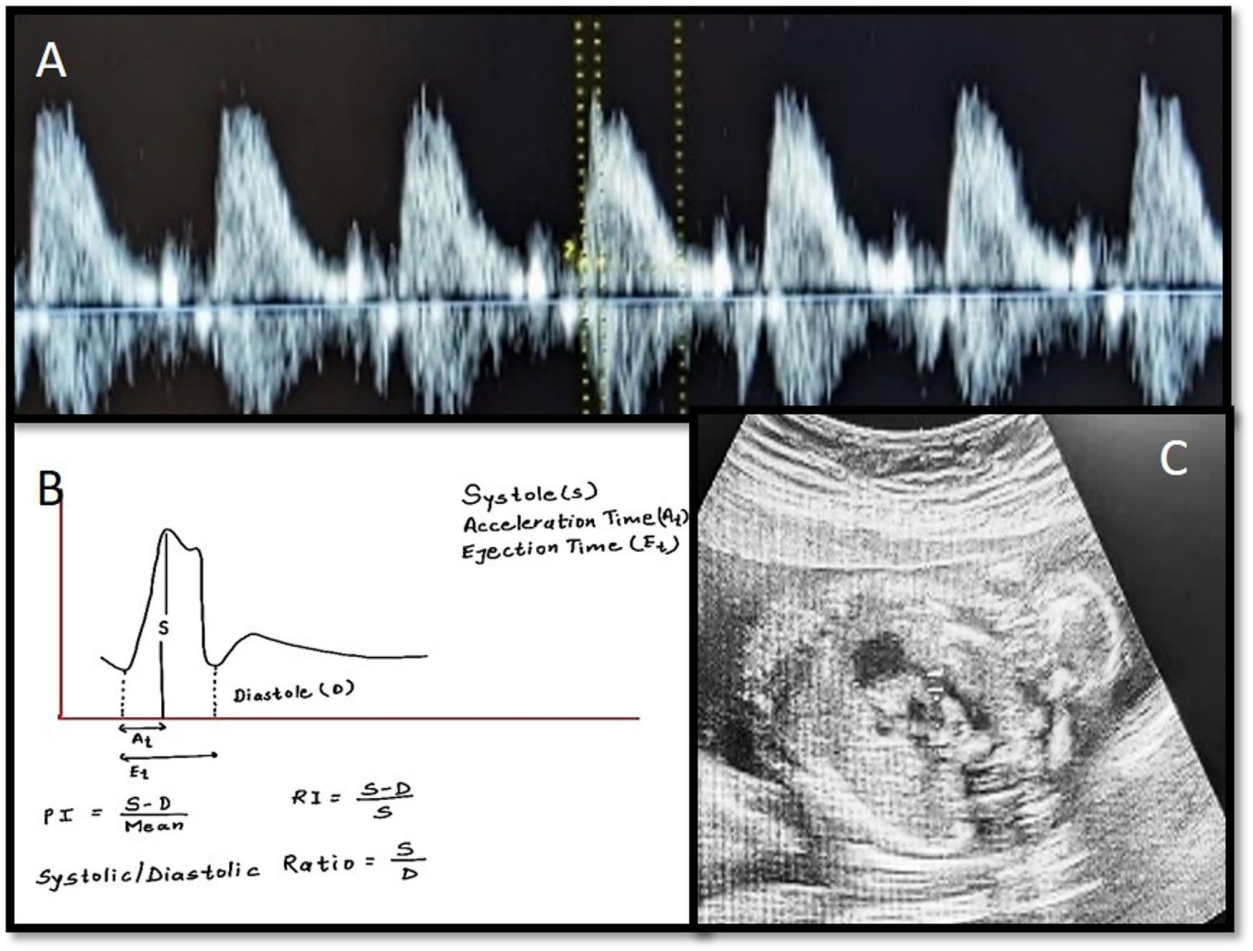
a Pulmonary artery wave form with classical spike (first peak) and gradual declining slope (spike and dome pattern). b Calculation of Doppler values c) sample ate is placed at mid pulmonary trunk
